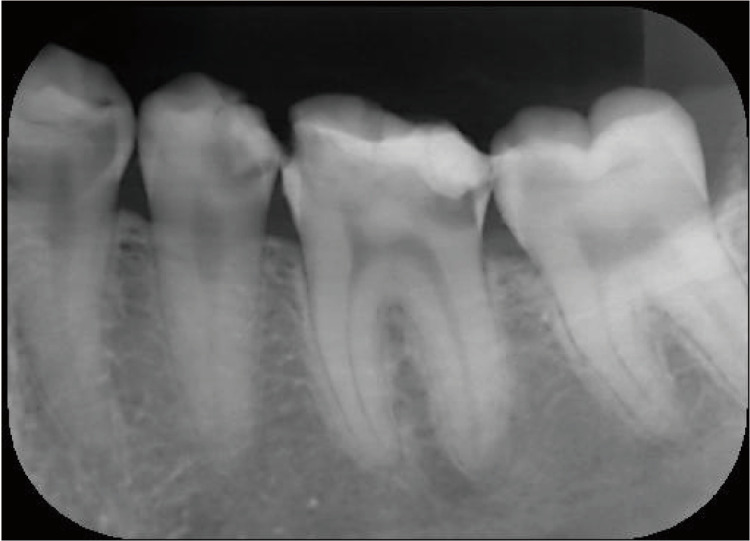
Dental radiograph of #45, #46, and #47.
